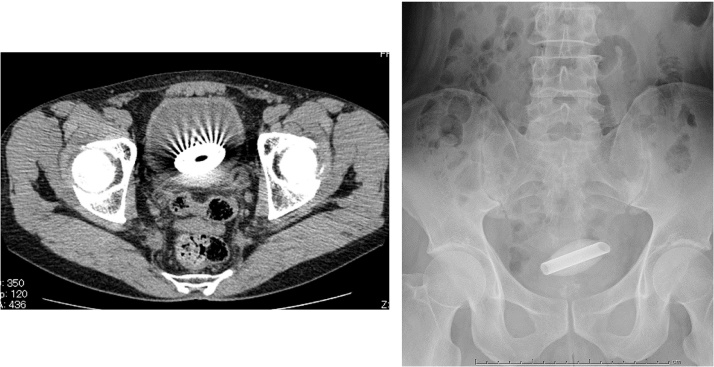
CT and KUB images of foreign body. A foreign body with an elliptical shape was found in the bladder. No mucous injury was observed.
